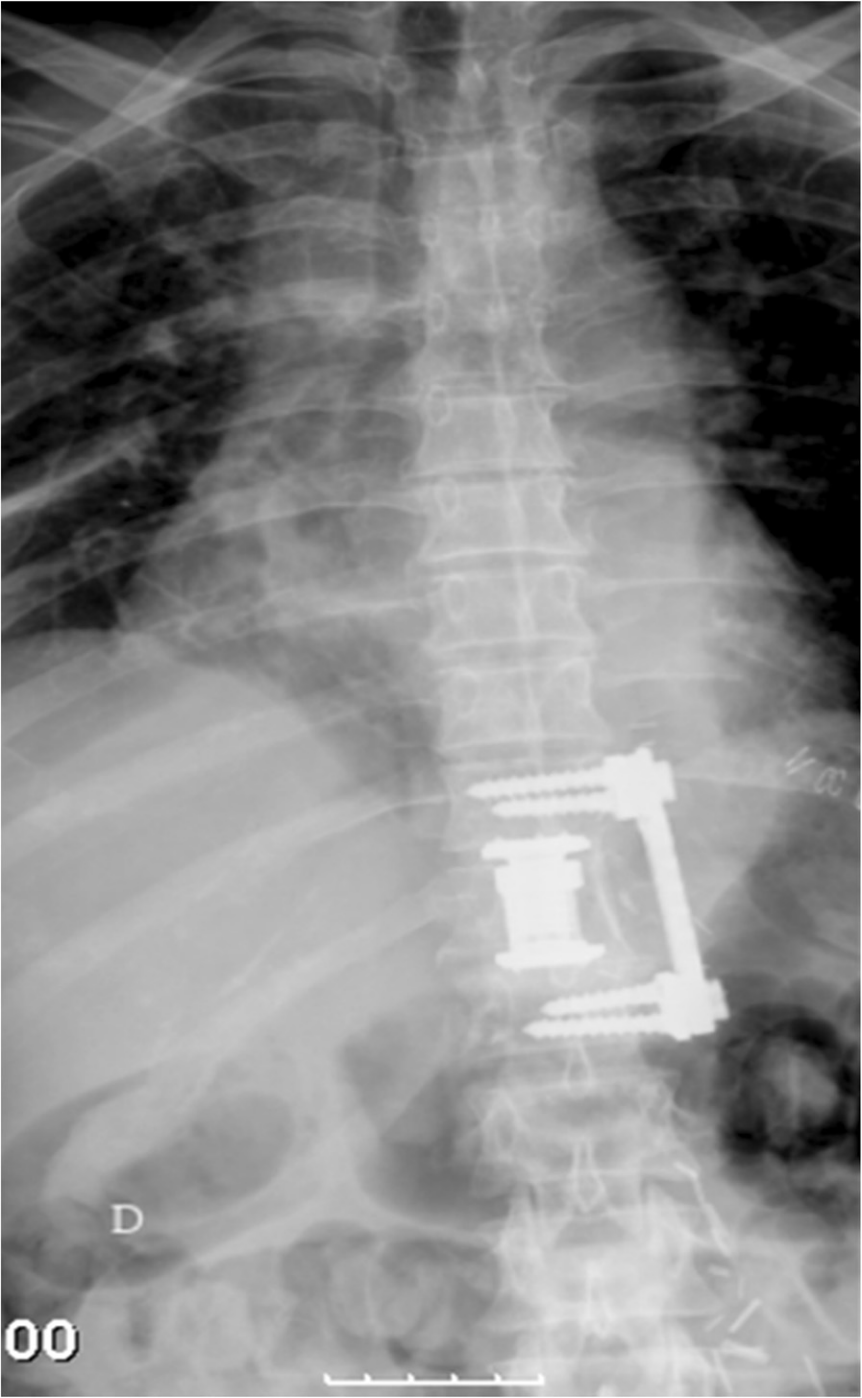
Post Op x-ray after corpectomy, replacement with an interbody spacer, autologous graft and a lateral plate.
